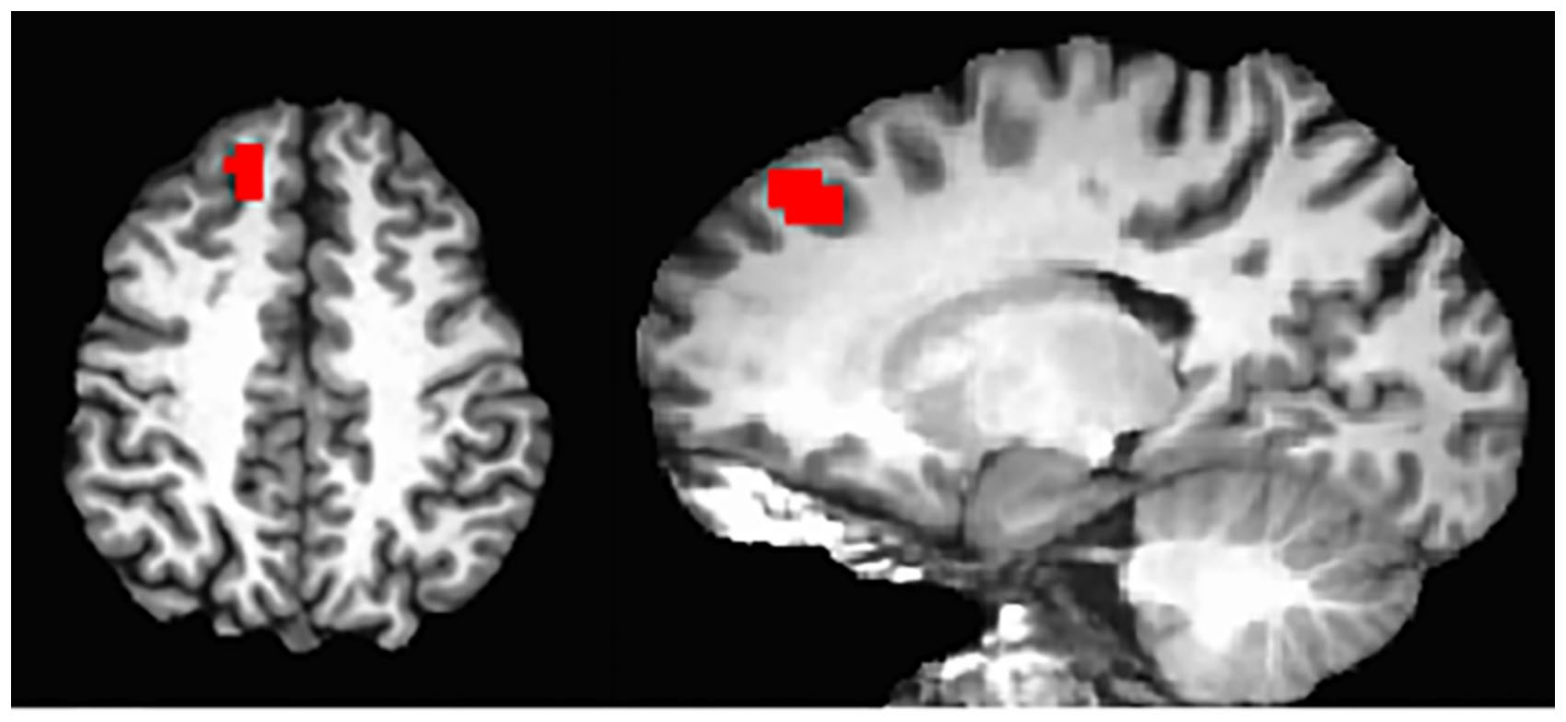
DMN connectivity covaried with RBANS total scores. Whole-brain analyses identified the regions where the resting-state functional connectivity seeded at posterior cingulate cortex is significant as well as covaried with the cognitive performance indexed by the RBANS total scores across all participants (*p*_corrected_ < 0.05), overlaid on an individual participant’s MRI. The region is a dorsal and medial portion of the PFC centered at the Talaraich coordinates of X = −16, Y = 38, Z = 41.
